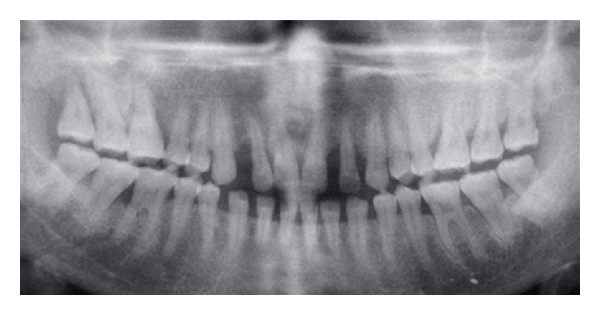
Orthopantomogram of the patient demonstrating generalized distribution of bone loss.
